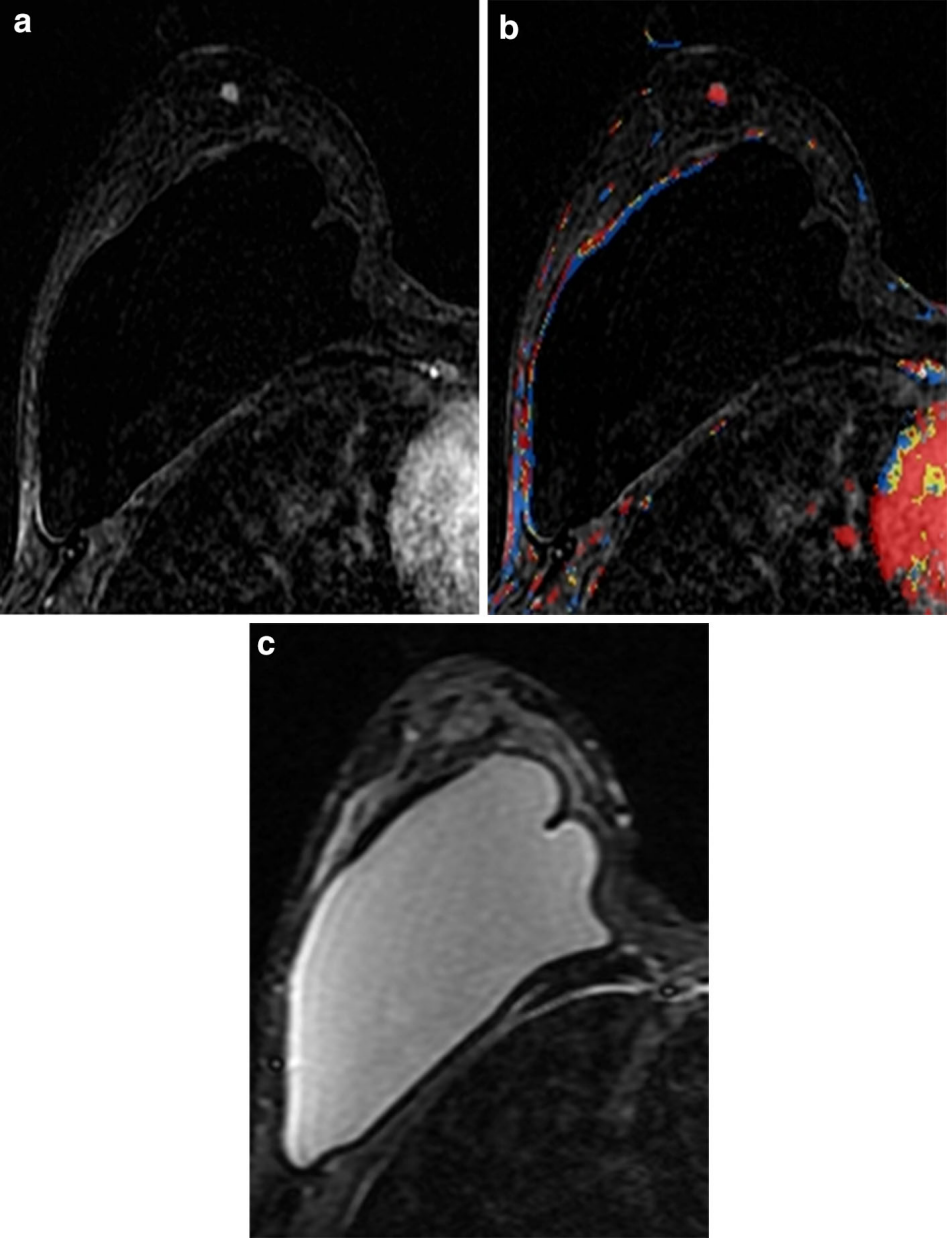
Focus with the absence of high signal on T2 sequence. a Postcontrast subtraction T1-weighted image shows a unique 0.4-cm focus with washout delayed kinetics (b) and the absence of high signal on fat-saturated T2-weighted image (c). Because this focus was new, it was assessed as probably benign, BI-RADS 3. Follow-up examination 6 months later showed increase in size of the focus; therefore, biopsy was recommended. MRI-guided wire localization was performed of this focus and surgery yielded invasive ductal carcinoma. For foci with washout kinetics and the absence of high T2 signal, biopsy should be considered
